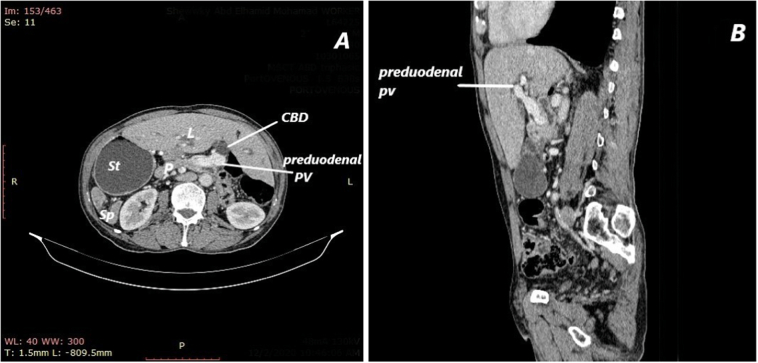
A: Computed tomography during portal phase shows heterotopic location of the liver, the stomach and the spleen The common bile duct is dilated. There are multiple small spleniculi, L: liver; St: stomach; Sp: spleen; P: pancreas. PV: portal vein. B: sagittal section showing preduodenal portal vein.
